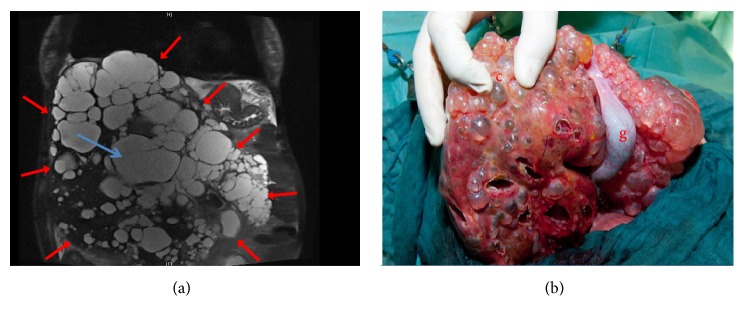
Well-perfused liver cysts (blue arrows) are highlighted by contrast medium (liver size marked with red arrows, preoperative computed tomography scan, early venous phase). Explanted cyst liver (b): gall bladder, g; cysts, c.
